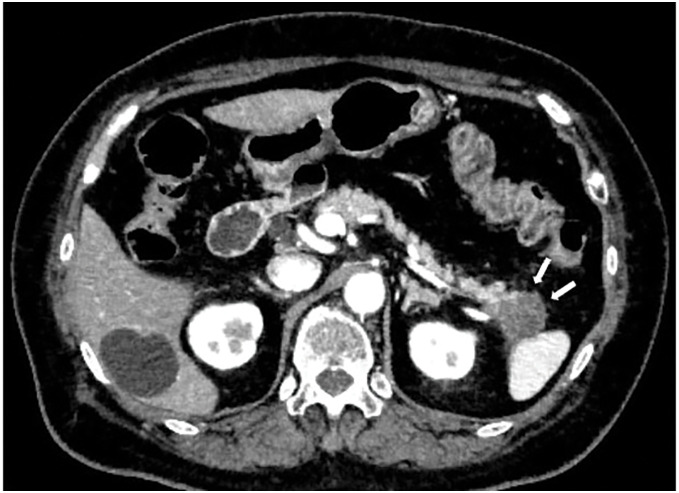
Contrast-enhanced CT showed a hypovascular tumor, 32 mm in diameter, in the pancreatic tail.
